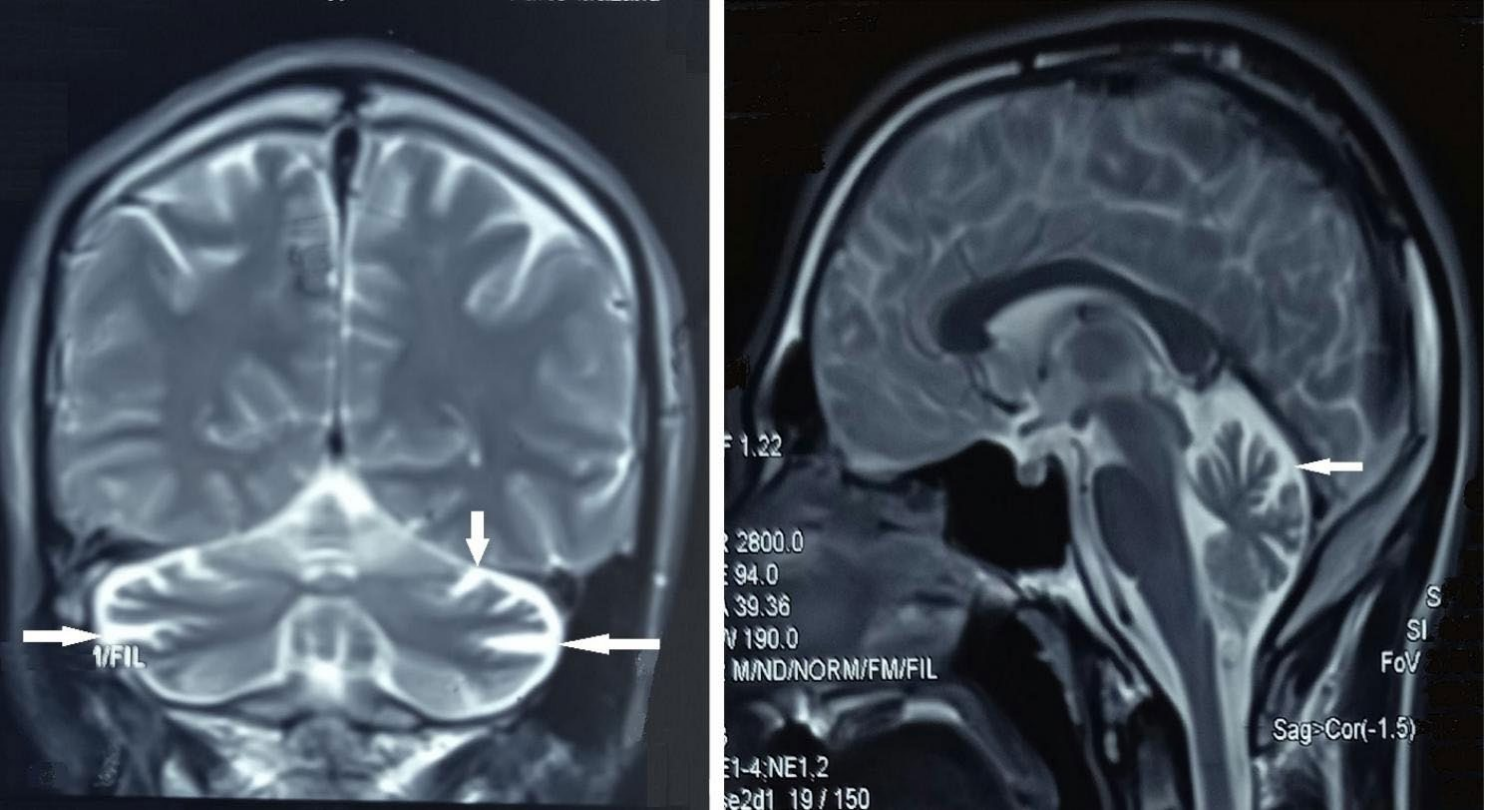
Brain MRI of patient IV.I showing cerebellar atrophy (arrows)
